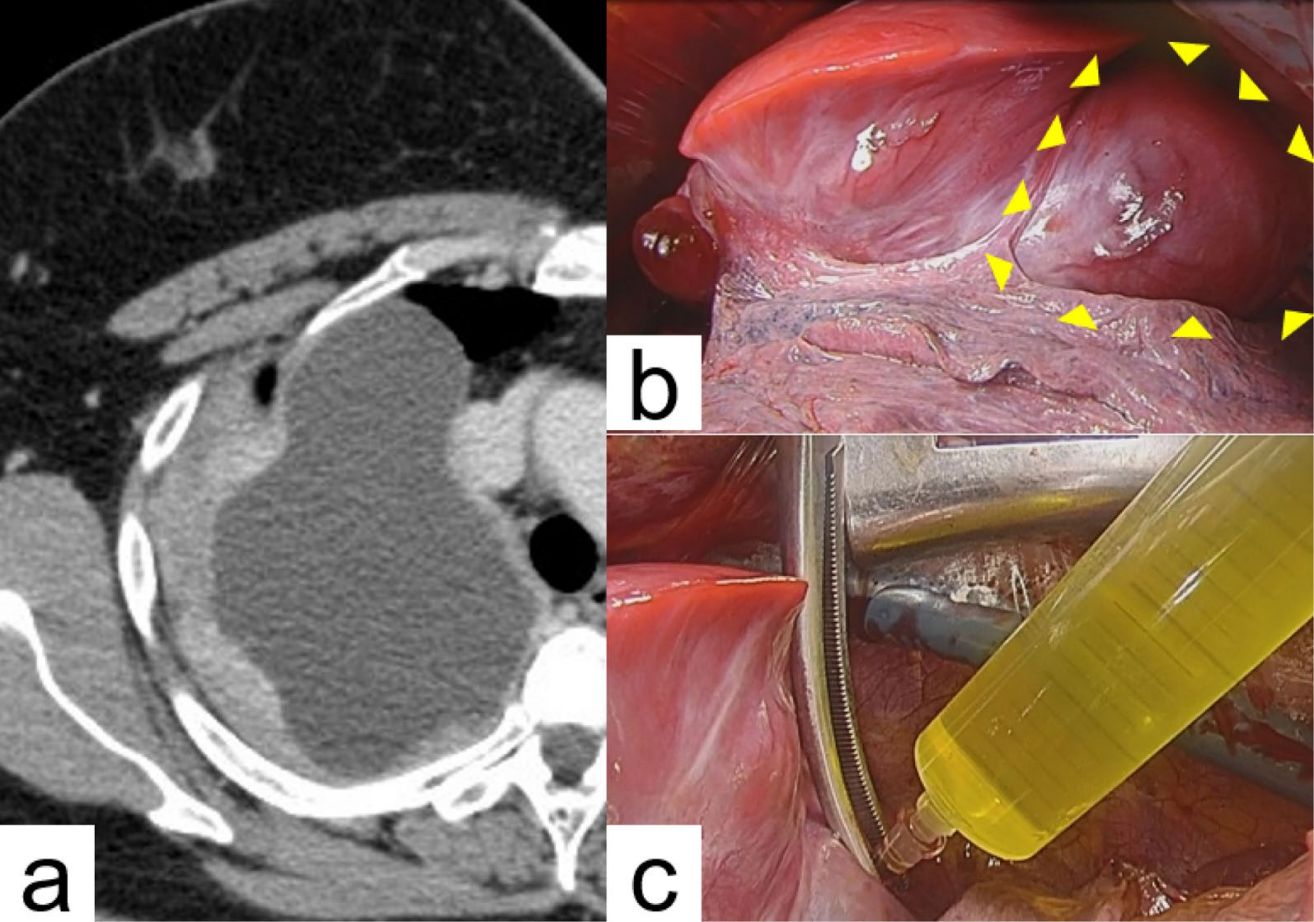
Preoperative computed tomography and intraoperative findings of rapid enlarged tumor in the right upper lobe. a The right upper lung lesion, especially cystic component, increased in size to 120 mm over a 7-month period. b The cystic component of the tumor, which had a dark-red surface, occupied most right upper lobe volume (yellow arrowheads). c Approximately 330 ml of serous fluid was egested from the tumor
